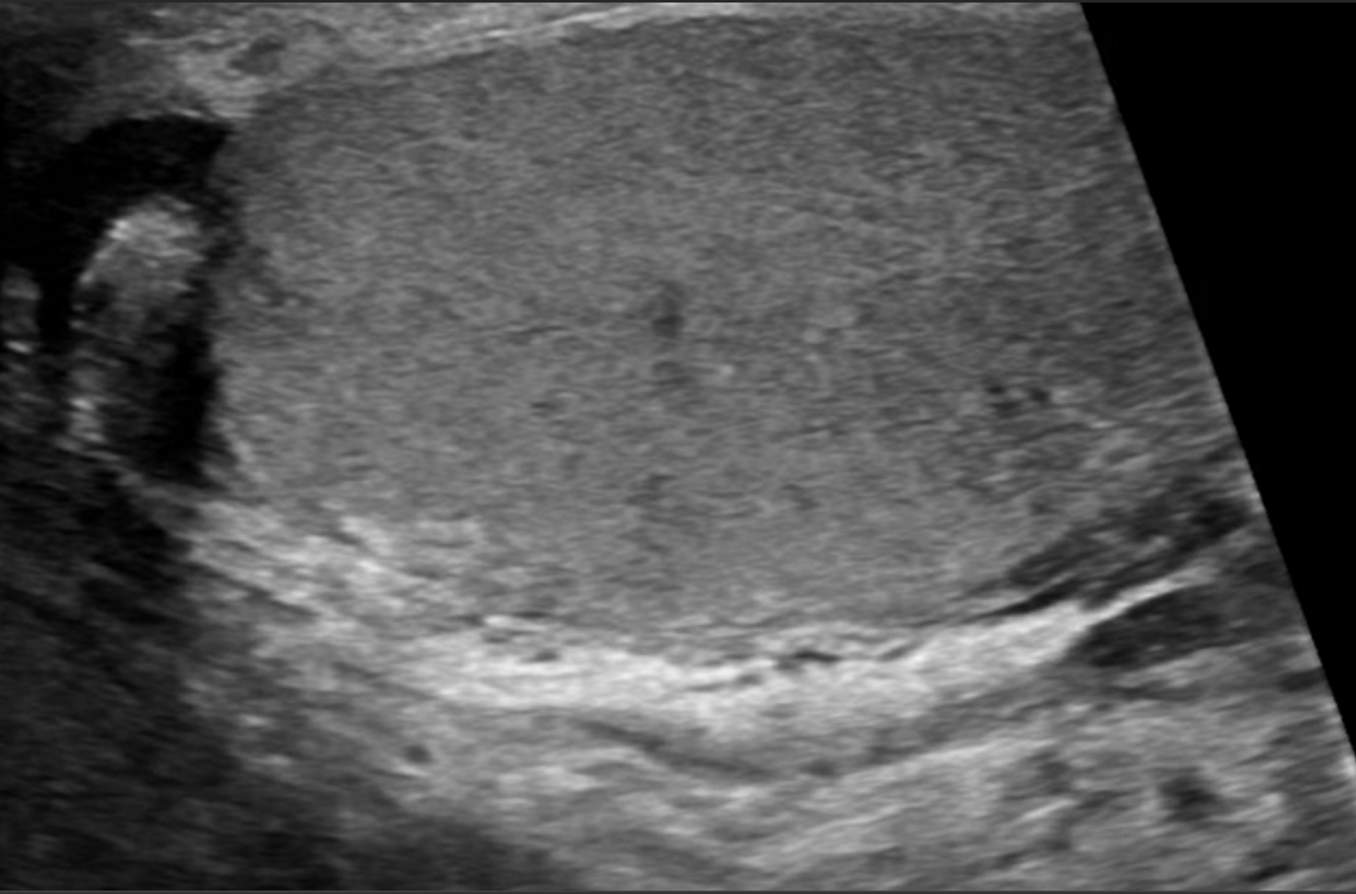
Ultrasound of scrotum & right testicle
